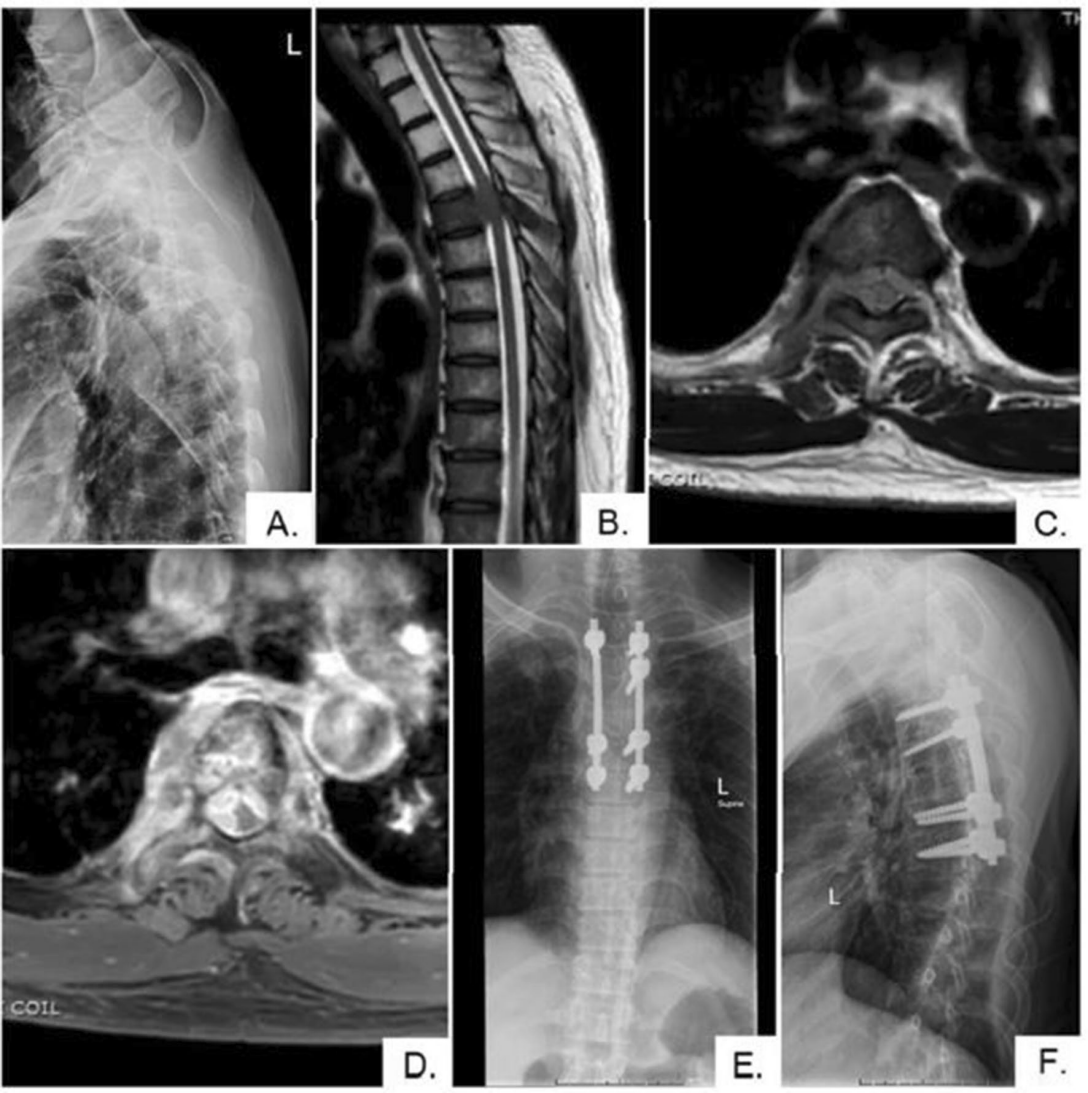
A 57-year-old male patient with tonsil cancer (case No. 14). (A, B) Lateral plain radiograph and sagittal T2-weighted magnetic resonance image (MRI) showing the mild collapse of the T5 vertebra and bone marrow involvement in the vertebral body due to metastases—extending posteriorly into the spinal canal compressing the spinal cord. (C, D) Axial T2-weighted and T1-weighted contrast-enhanced MRI confirm the tumor extension into the spinal canal. (E, F) Anteroposterior and lateral plain radiograph obtained 3 months after posterior instrumentation and decompression without anterior reconstruction, showing no implant-related complication. Please note that the tumor was already involved at the right T4 pedicle at the time of surgery. Thus, the surgeon was unable to insert the pedicular screw securely.
